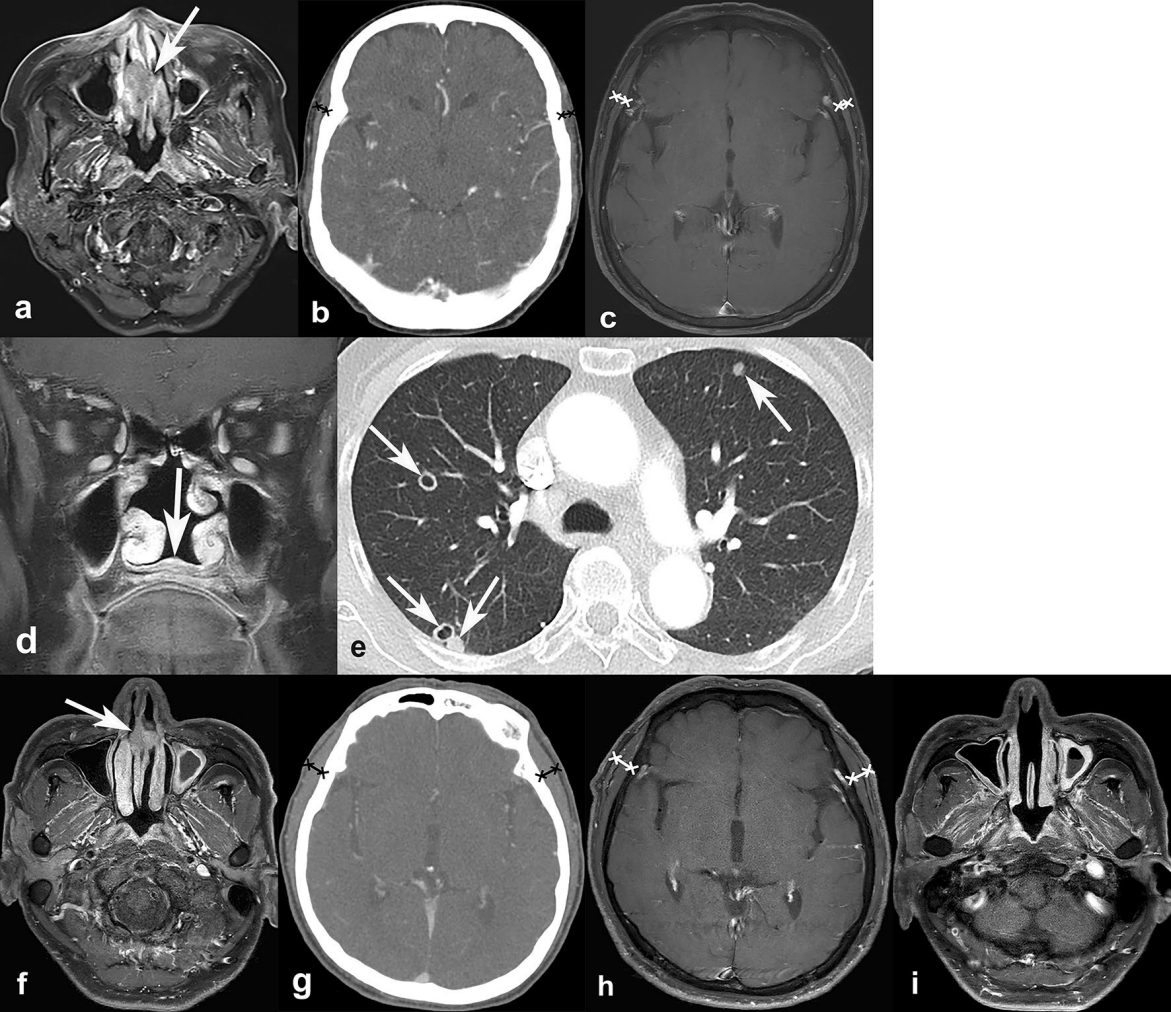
Representative cases of temporalis muscle thickness (TMT) on CT and MRI, and its association with progression-free survival. A 72-year-old female was diagnosed with nasal cavity cancer (cT2N0M0) (a, arrow). Her baseline TMT was measured as 4.22 mm on CT (b) and 4.82 mm on MRI (c). During the follow-up, she developed local tumor recurrence at nasal floor (d, arrow) and multiple pulmonary metastases (e, arrows). Another 66-year-old male was diagnosed with nasal cavity cancer (cT1N0M0) (f, arrow). His baseline TMT was 7.32 mm on CT (g) and 9.43 mm on MRI (h). After surgery, he did not show any progression during the 30 months of follow-up (i).
